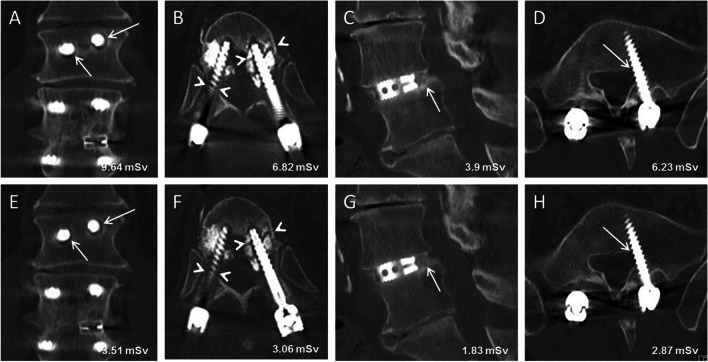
Standard 120 kV CT (A-D) and corresponding 140 kV tin-filtered low-dose CT (E–H) in 4 different patients after lumbar instrumentation. 60-year-old male (A and E) with bilateral loosening of pedicle screws at level L1 (arrows). 68-year-old male (B and F) with bilateral pedicle fracture at level Th12 (arrowheads). 80-year-old male (C and G) with osseous fusion posterior to the cage at level L4/5 (arrows). 27-year-old male (D and H) with left pedicle screw traversing lateral recess at level S1 (arrows)
